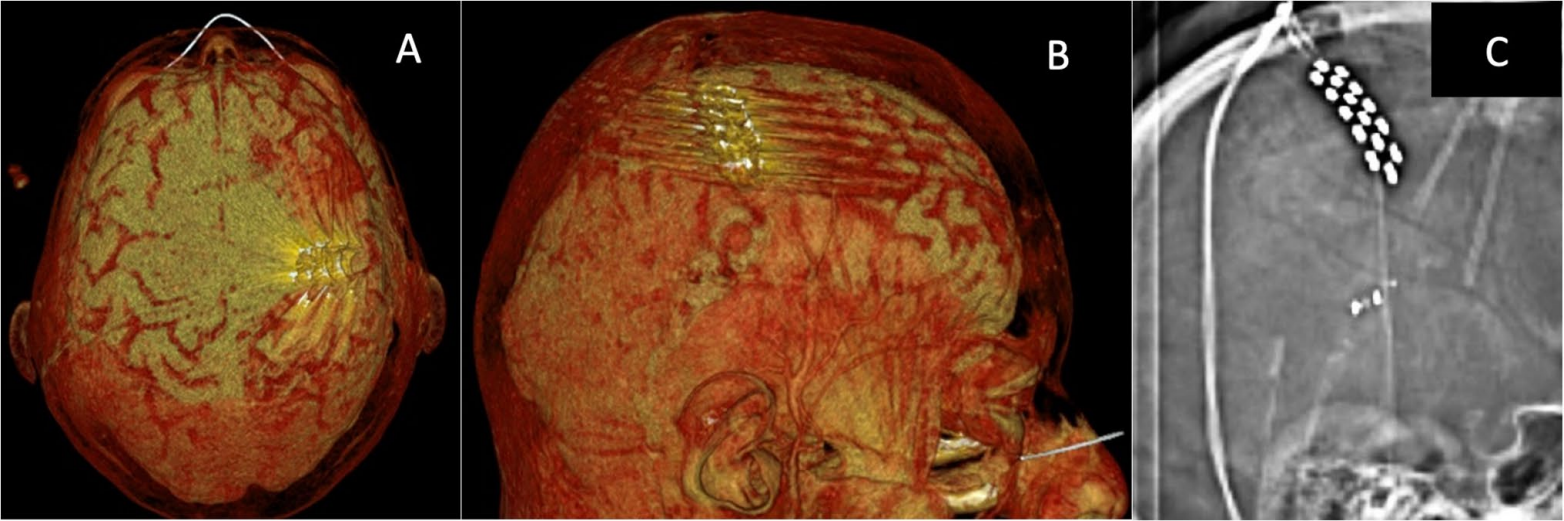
Postoperative electrode position in the computed tomography (CT) scan. A 3D reconstruction of the CT data set (syngo.via software, Siemens Healthinears) with depiction of the electrode positioned over the right motor cortex from a superior-inferior perspective. B 3D reconstruction of the CT data set (syngo.via software, Siemens Healthinears) with depiction of the electrode positioned over the right motor cortex from a lateral perspective. C Localizer (topogram) from the CT data set with depiction of the implanted electrode encompassing 16 stimulation contacts
